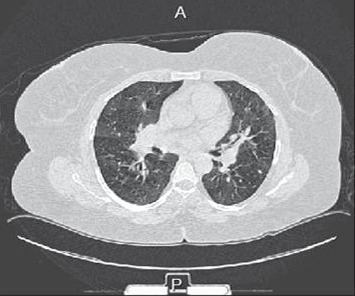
A high-resolution computed tomography (HRCT) scan of the patient chest showing a bilateral micronodular interstitial pattern with hilar adenopathy.
